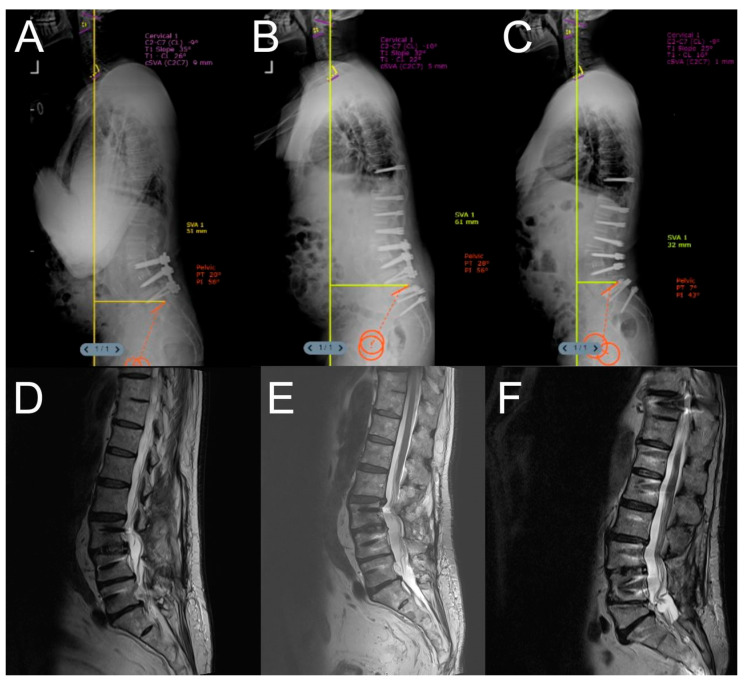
A patient who had previously undergone L3–4 interbody fusion and L3–5 stabilization for spondylolisthesis presented with difficulty looking forward and increased kyphosis. (A) Preoperative lateral X-ray image. (B) Lateral X-ray image after the first-stage surgery. (C) Lateral X-ray image after the second-stage surgery. (D) Sagittal MRI image after the initial L3–5 stabilization. (E) Preoperative sagittal MRI image showing proximal junctional kyphosis and Pfirrmann grade 4 intervertebral disc degeneration. (F) Postoperative sagittal MRI image after the second-stage surgery. Due to the patient’s osteoporosis (T-score = −2.5 preoperatively, T-score: −1.5 before the two-stage surgery), a two-stage surgical approach was adopted. Significant improvement was observed in both clinical findings and spinopelvic parameters in the postoperative period.
